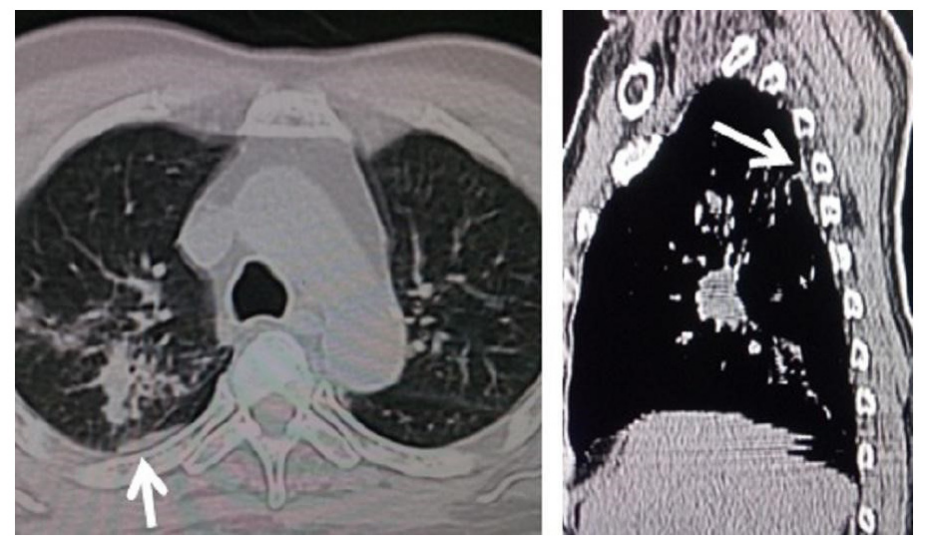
Repeat CT image revealing good resolution in the size of the pleural based nodule during the follow up at 1 month.
